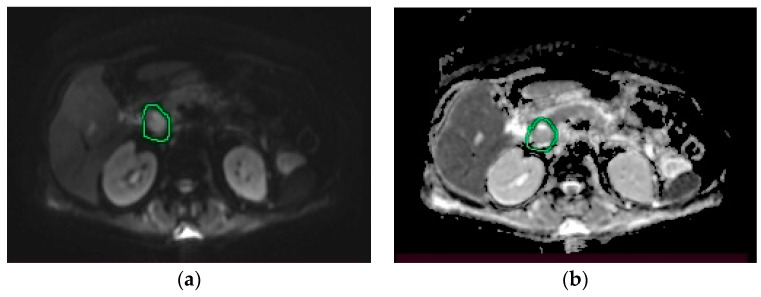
MRI. (a) Diffusion-weighted imaging (DWI) sequence: hyperintense cephalopancreatic lesion; (b) apparent diffusion coefficient (ADC) sequence: hypointense cephalopancreatic lesion.
